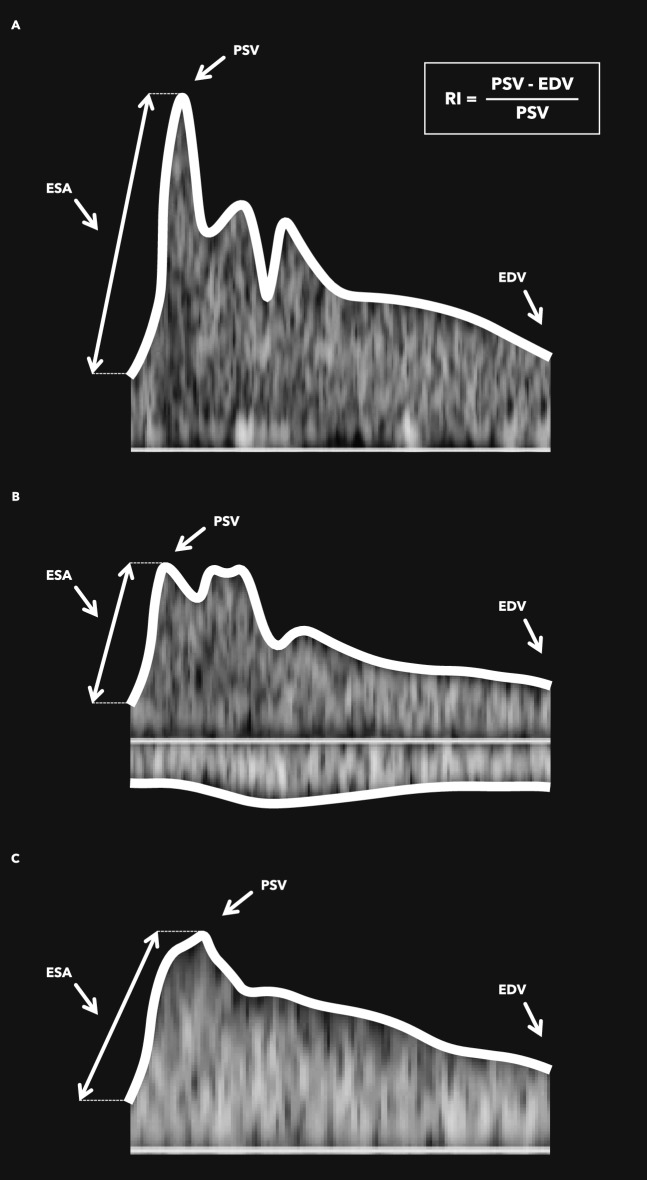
Doppler waveforms of the ophthalmic vessels. A Doppler of the ophthalmic artery showing a higher PSV and ESA compared with the central retinal artery (B) and short posterior ciliary artery (C). There is also a greater difference between PSV and EDV resulting in a higher RI in the ophthalmic artery compared to the other vessels in the orbit. B Doppler of the central retinal artery, which also portrays the central retinal vein, as these vessels have an analogous trajectory inside the optic nerve sheath. The central retinal vein waveform is located below the reference line, due to the inverse flow direction of venous drainage. C Doppler of the short posterior ciliary artery showing slower velocities, resistance, and acceleration. EDV, end-diastolic velocity; ESA, early-systolic velocity; PSV, peak-systolic velocity; RI, resistive index
